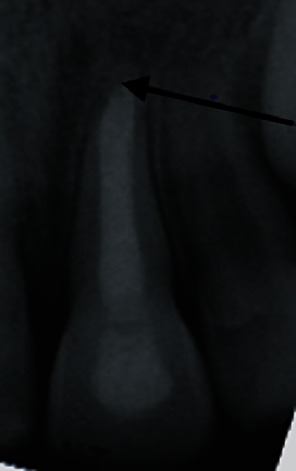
Posteroanterior radiograph of tooth #21 four months after obturating with MTA.
